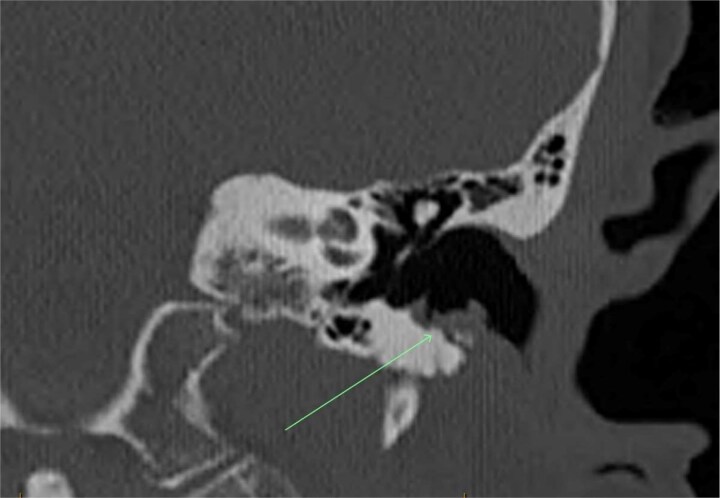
Case A—coronal CT scan of left ear canal, arrow demonstrating area of inferior canal erosion with overlying lobulated soft tissue.
